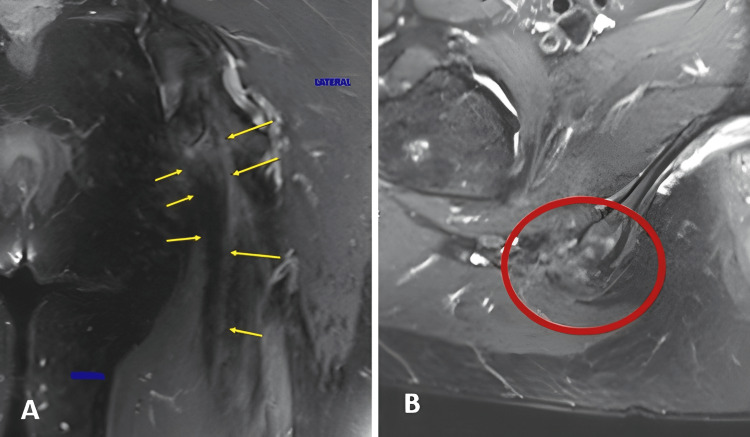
Postoperative MRI (A) Postoperative coronal T2 MRI showing the reinserted hamstring tendons at the ischial tuberosity (yellow arrows); (B) Postoperative axial T2 fat-suppressed image demonstrating the hamstring allograft reinsertion (red circle). Image Source: Faculty of Medical Science and Health, Pontifical Catholic University of São Paulo; Used with Permission
